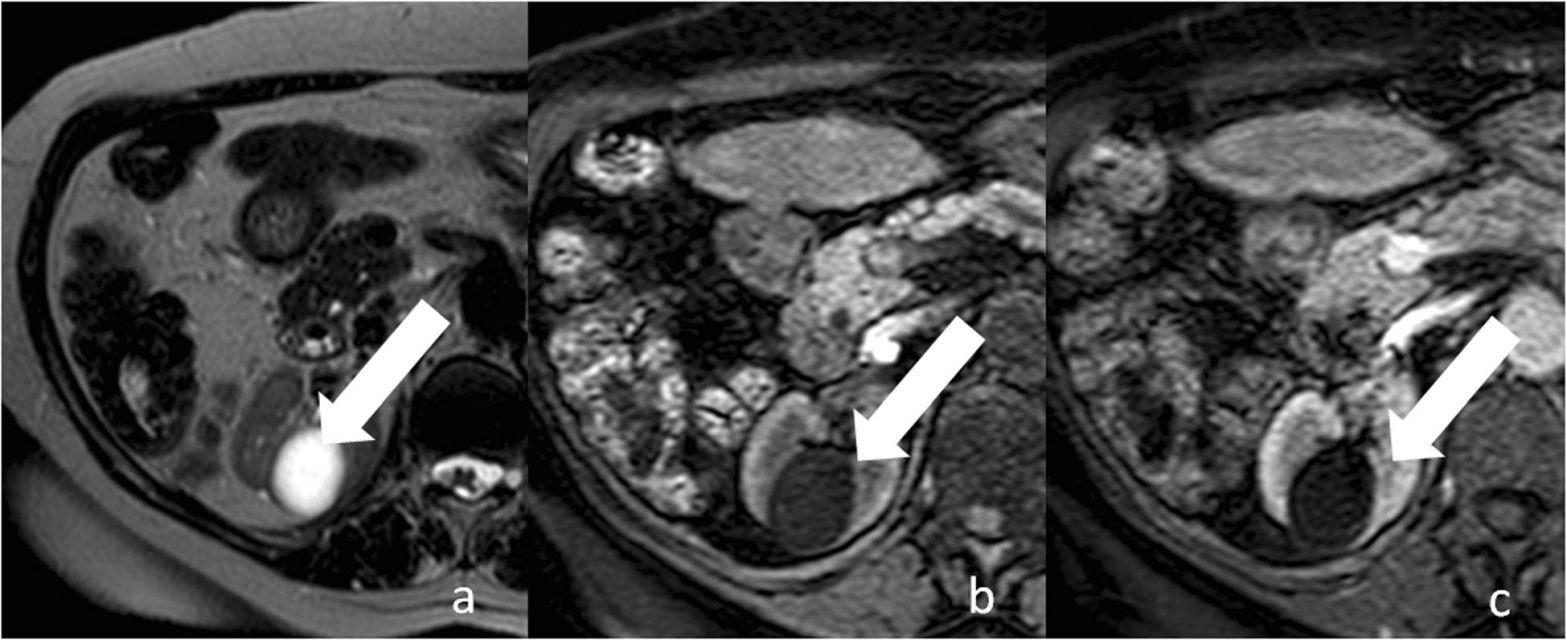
Bosniak category I renal cyst. a Axial T2-weighted MR image shows a lesion (arrow) with strong hyperintensity and a thin wall. Corresponding axial non-enhanced (b) and contrast-enhanced (c) T1-weighted MR images show a hypointense lesion with a thin and non-enhancing wall
